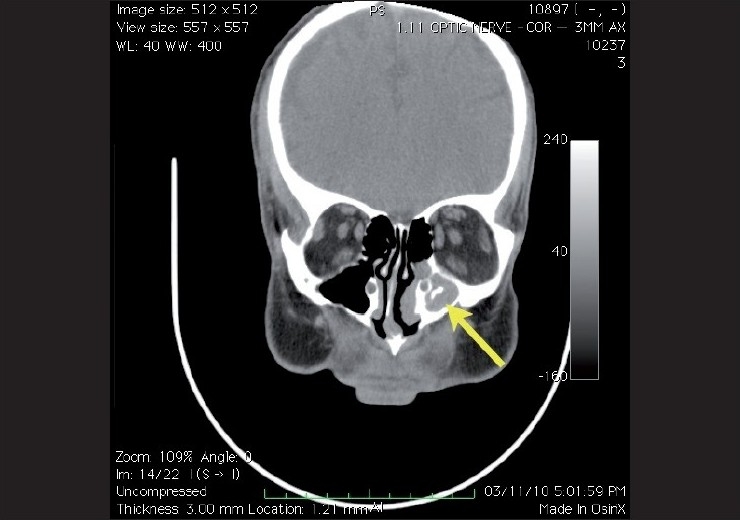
Coronal CT scan of the orbit at the mid orbit level showing normal optic nerves and extra ocular muscles. Note the left maxillary sinusitis (yellow arrow)
